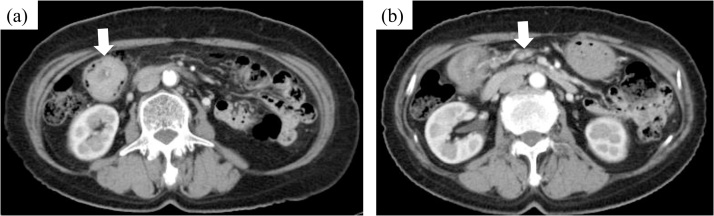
Contrast-enhanced computed tomography findings. (a) The tumor occupied the transverse colon. (b) Several regional lymph nodes were swollen.
